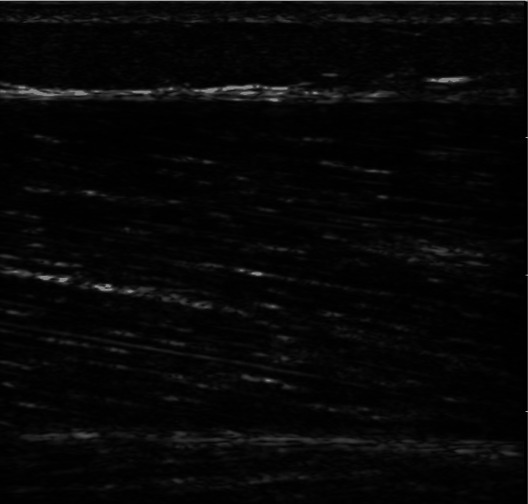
A typical ultrasound scan.
